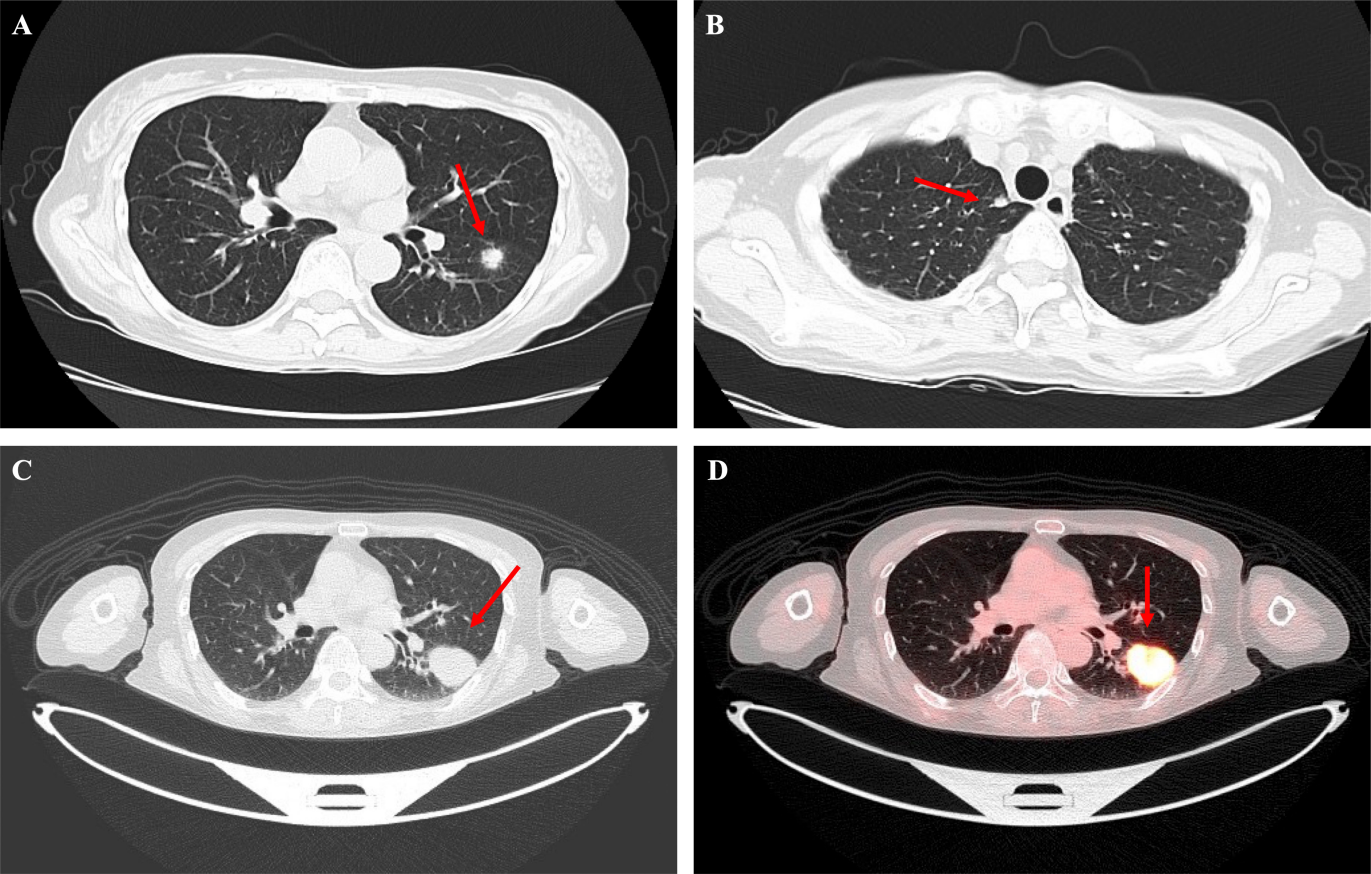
Chest computed tomography (CT) reveals a 12‐mm nodule in the left S6 segment (A) and multiple smaller nodules (B) (red arrows). Positron emission tomography/CT shows enlargement of the nodule to 36 mm (C), with high 18F‐fluorodeoxyglucose uptake (standardised uptake value max, 17.97) (D) (red arrows).
